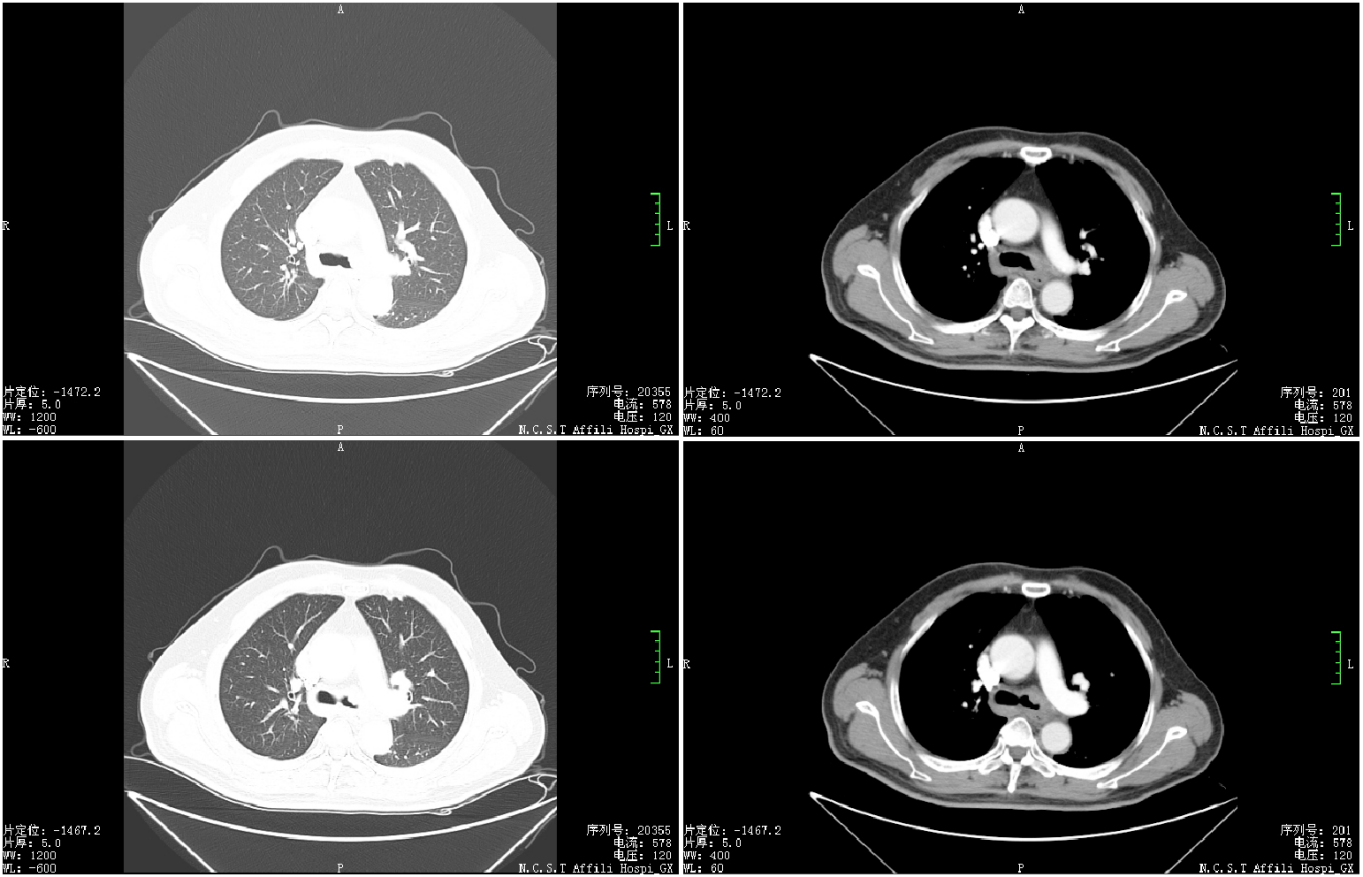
Chest computed tomography scan showing a left main bronchial mass.
